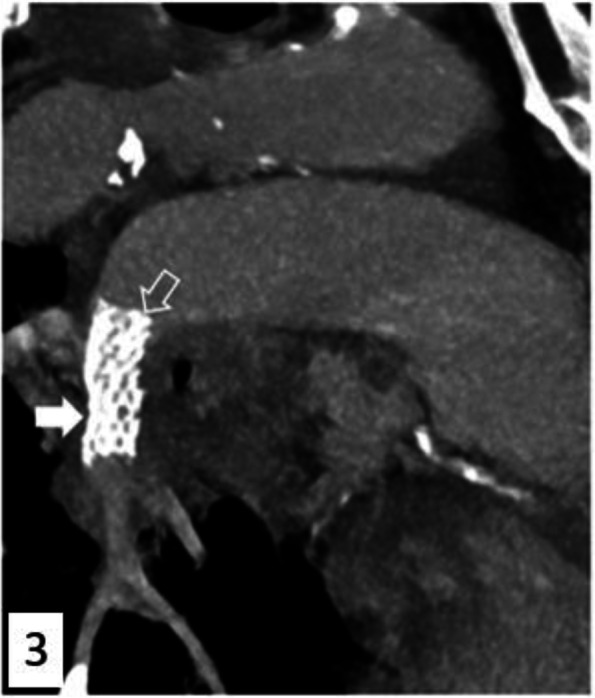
Postoperative computed tomography angiography after stent deployment (*empty arrow*) placement shows the exclusion of the pseudoaneurysm and the patency of downstream arteries. Sagittal MIP 10 mm reconstruction 80 keV 130 mAs Somatom X.Cite Siemens Healthineers Erlangen
